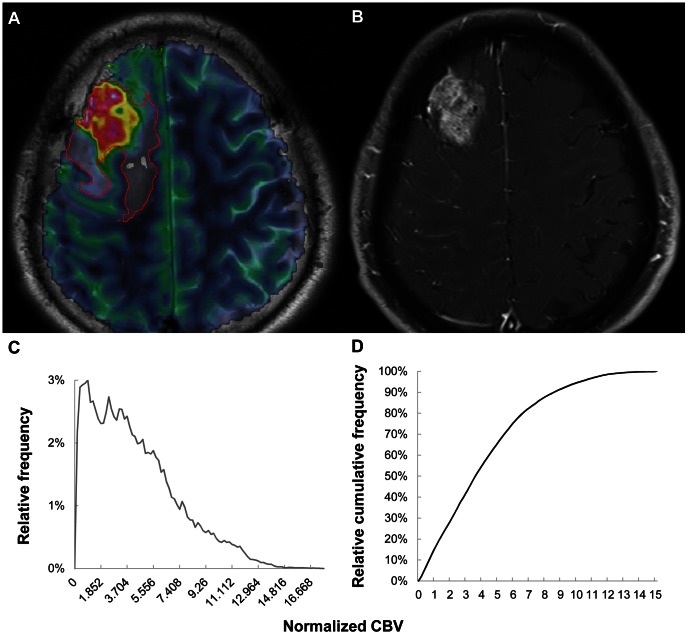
Images of a 50-year-old female with a grade IV glioblastoma. (A) ROI-drawn rCBV map overlaid on a T2-weighted image, (B) contrast-enhanced T1-weighted image, (C) histogram of nCBV and (D) cumulative nCBV histogram. The 99^th^ percentile value of the cumulative nCBV histogram was 12.489. ROI = region of interest; rCBV = relative CBV; nCBV = normalized CBV.
